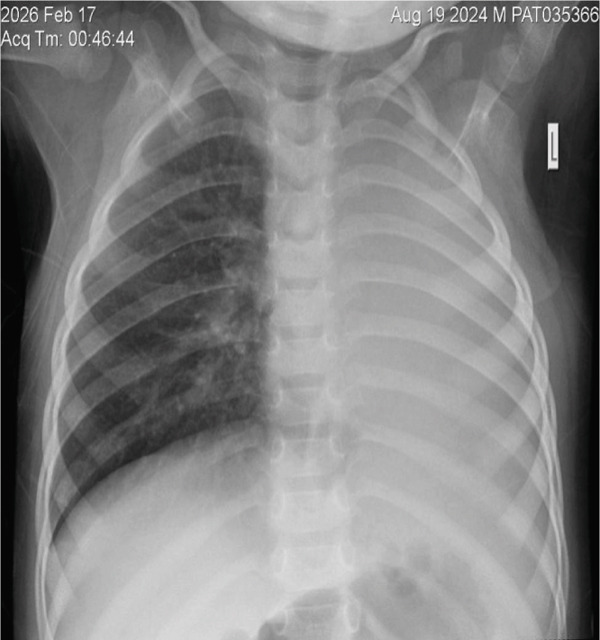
Chest radiograph demonstrating complete left lung collapse with ipsilateral mediastinal shift.
